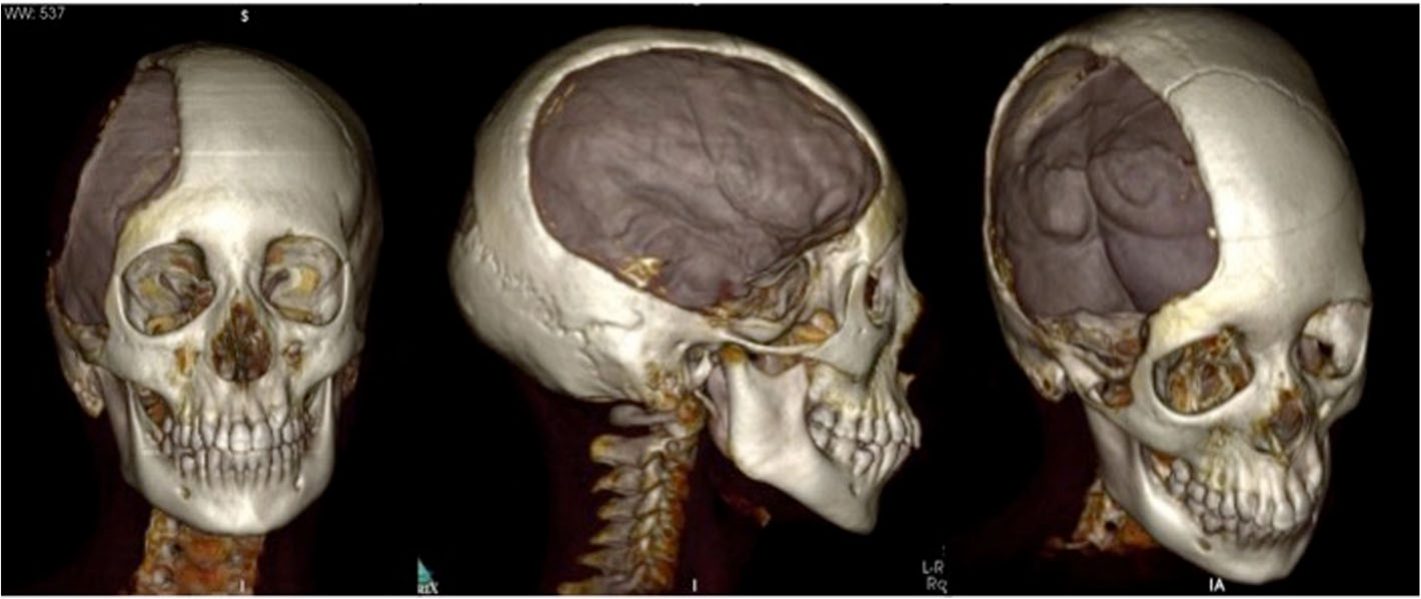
CT showing a 12 × 12 cm right cranial defect. The prosthetic design was done using a computer system (DICOM) to design and produce a customized prosthesis in acrylic resin
